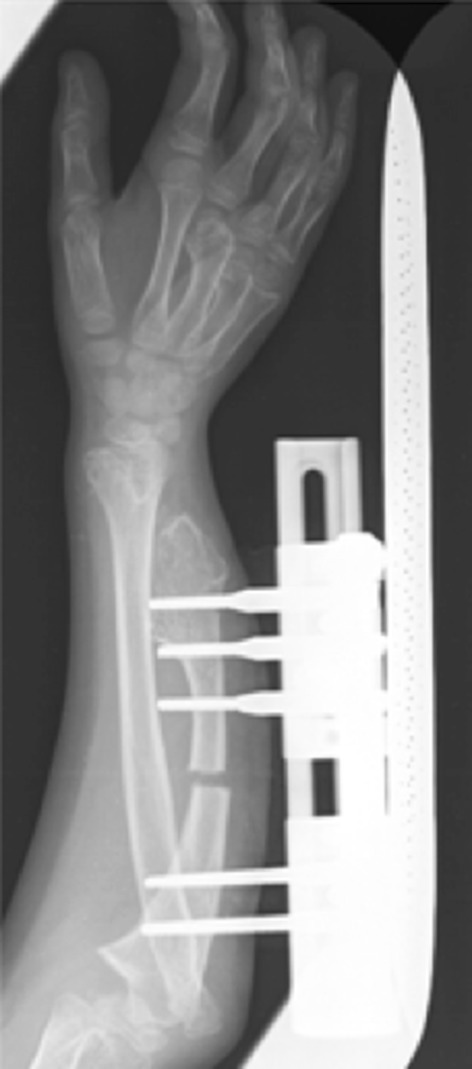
Anteroposterior radiograph of a forearm 15 days after surgery: Correct positioning of the external fixator, removal of exostosis, and osteotomy of the ulnar shaft
